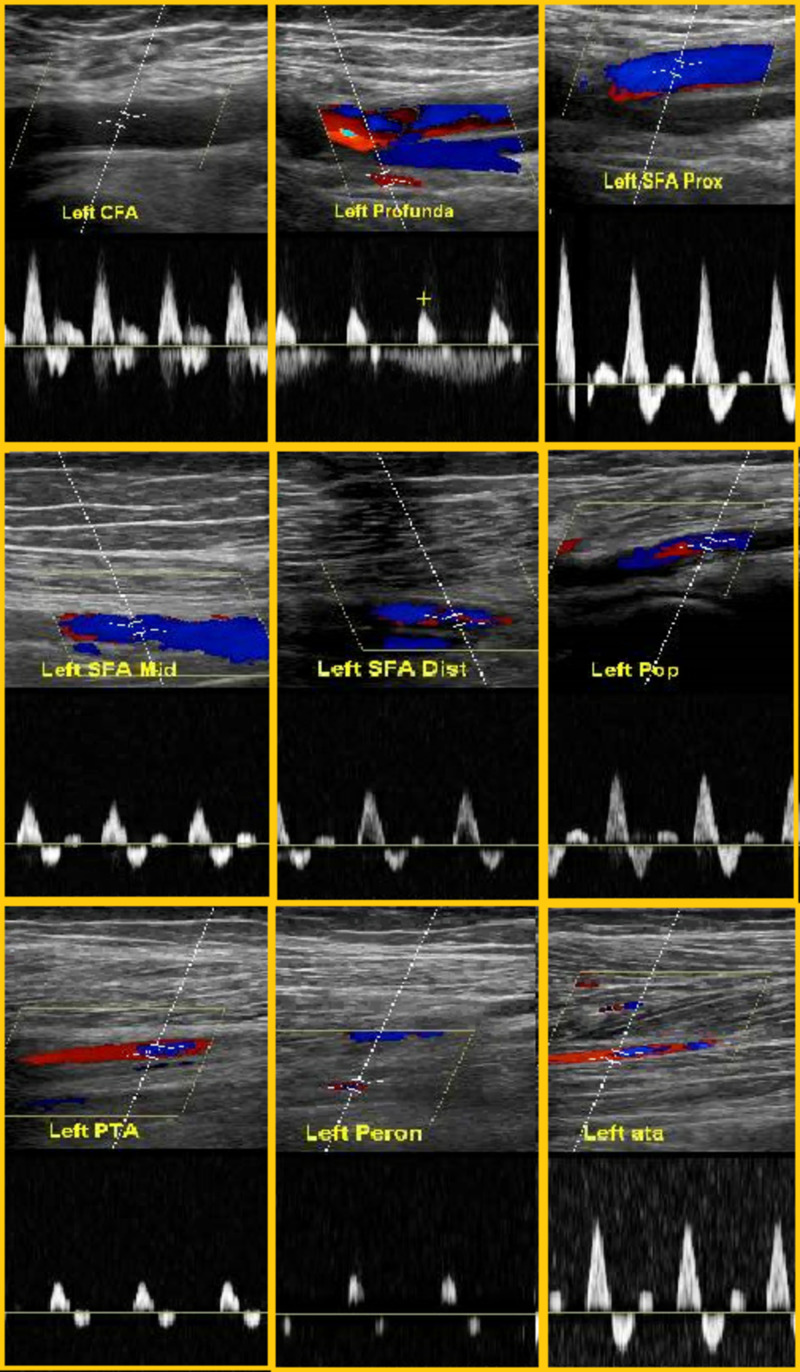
Arterial ultrasound of the left lower extremity with waveforms The arterial ultrasound of the left lower extremity did not show any occlusions. CFA, common femoral artery; SFA Prox, superficial femoral artery proximal; SFA Mid, superficial femoral artery mid; SFA Dis, superficial femoral artery distal; Pop, popliteal; PTA, posterior tibial artery; Peron, peroneal; ATA, anterior tibial artery
